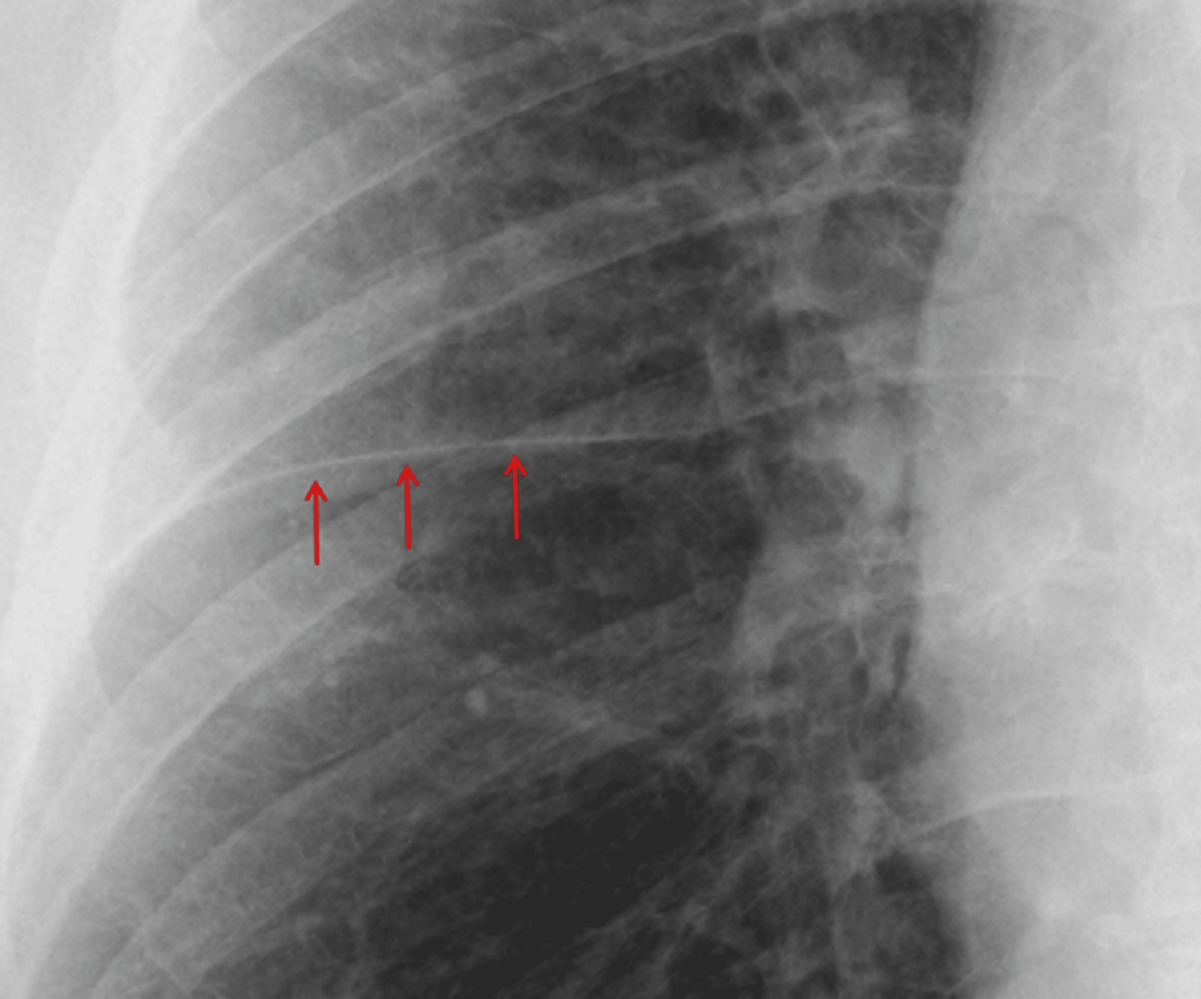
Post-angiogram CXR showing Kerley B lines in the lower zone Accentuation of the right horizontal fissure (red arrows) indicates the presence of interstitial fluid, consistent with Kerley B lines. CXR, chest X-ray
